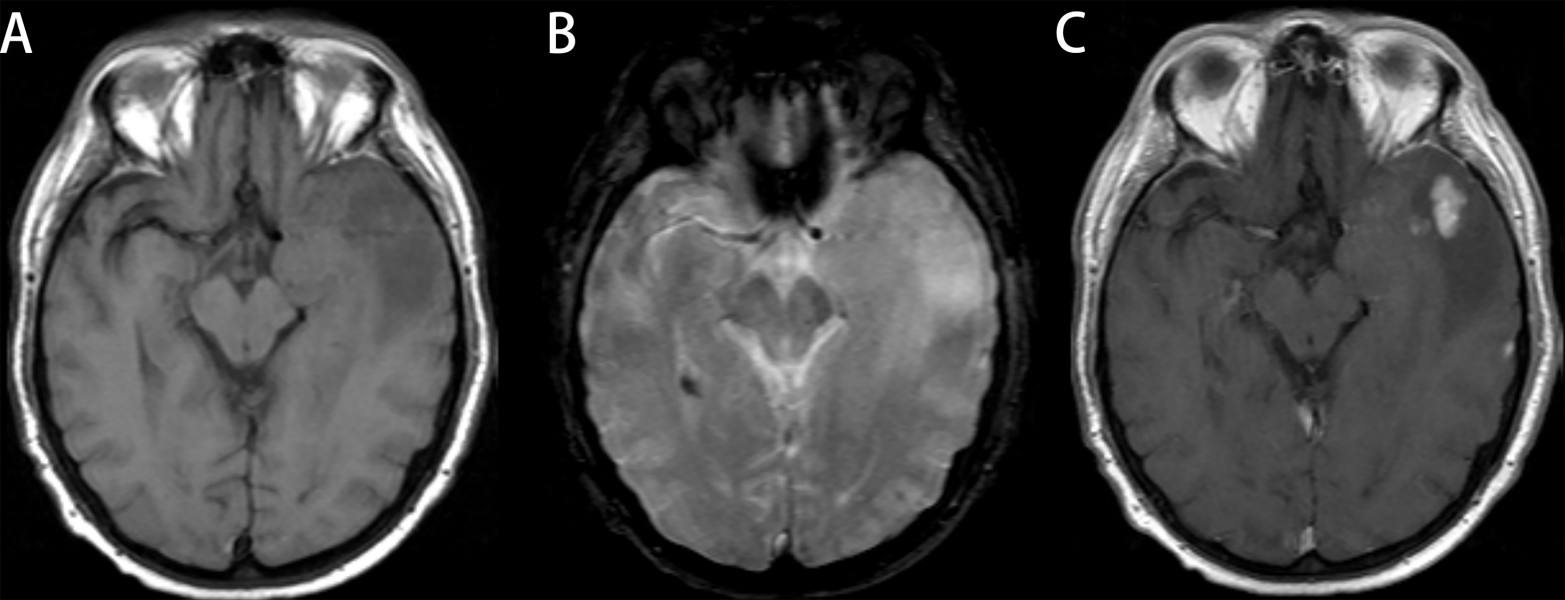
Glioblastoma (GBM) in a 78-year-old man with a license named TCGA-76-6193 (TCGA-76-6193). (A) Axial T1-weighted MR images demonstrate an area of high signal intensity in the left temporal region with adjacent edema. (B) Axial T2-weighted MR image reveals slightly high signal in the lesion. (C) Axial T1-weighted MR image with contrast material showed significant and heterogeneous enhancement in the lesion. All three readers diagnosed the lesion as low-grade glioma (LGG). Radiomics predict_proba_GBM was 0.701 (cutoff value = 0.610), and the radiomics model diagnosed it as GBM.
